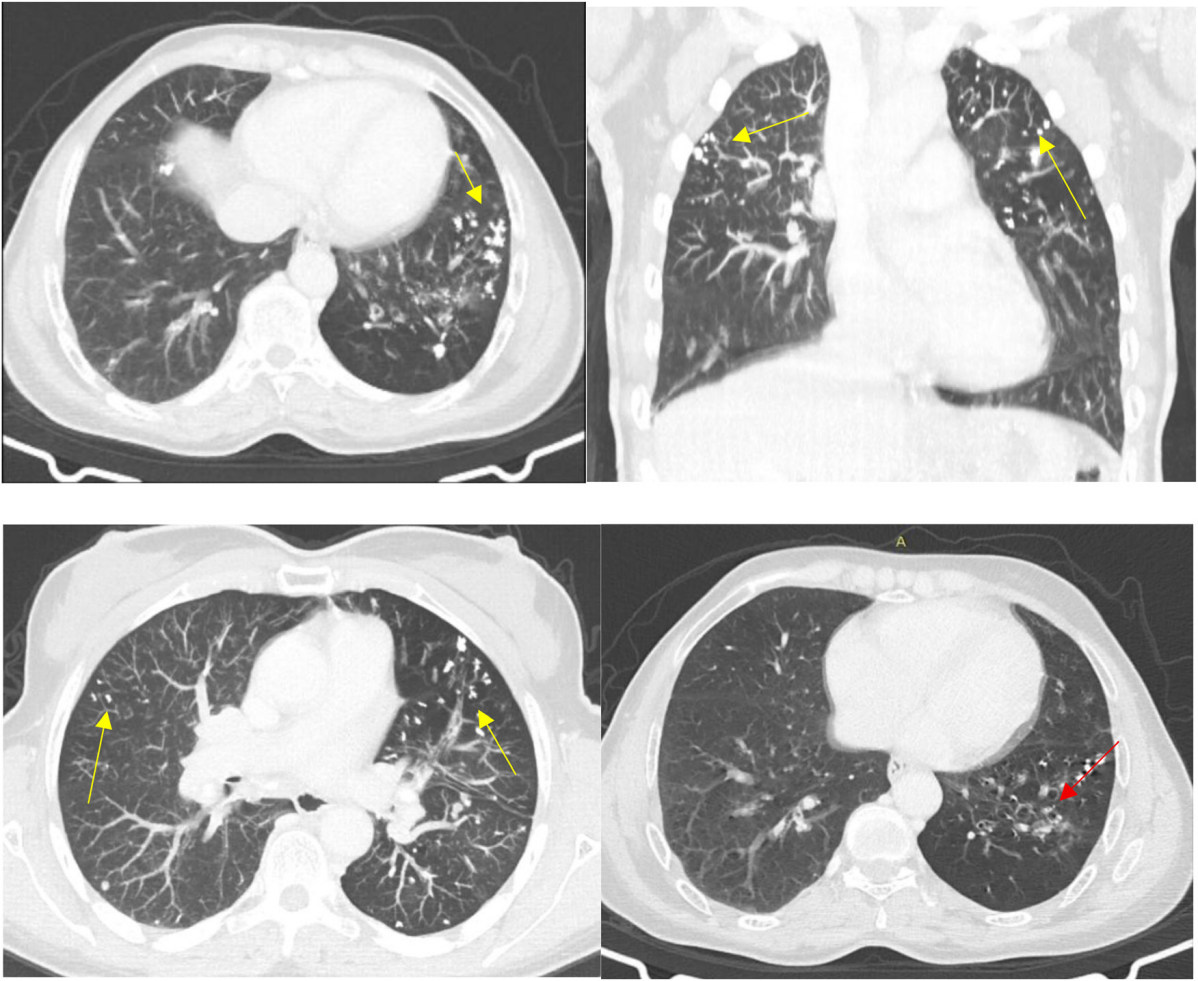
Axial and coronal CT images (lung window) demonstrating multiple predominantly upper-lobe calcified micronodules (yellow arrow) with centrilobular and perilymphatic distribution, associated with traction bronchiectasis (red arrow).
